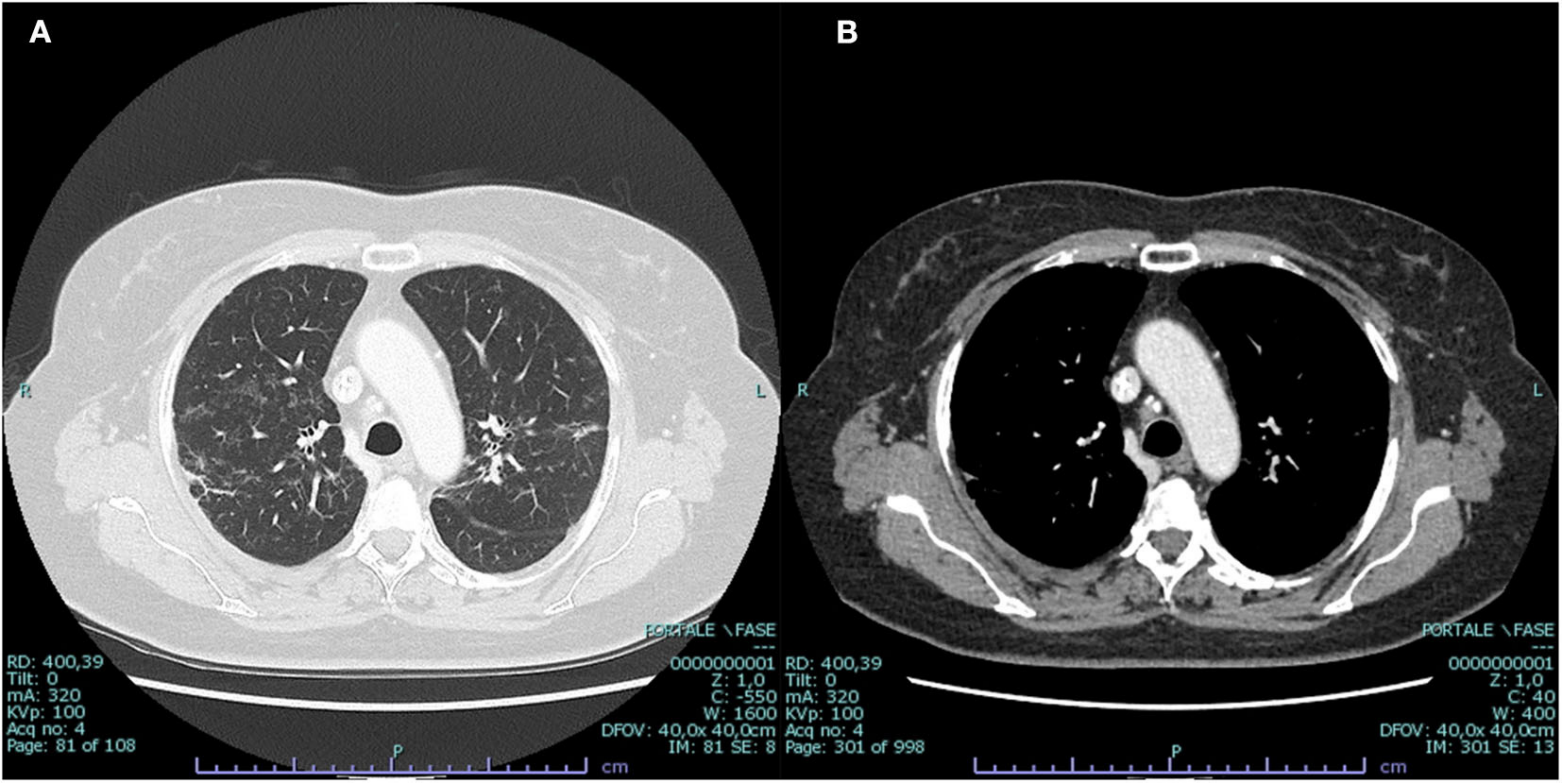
CT scan after treatment: remaining parenchymal fibrosis in upper lobes compatible with sarcoidosis grade IV (A), without mediastinal or extra-thoracic lymph node enlargement (B).
